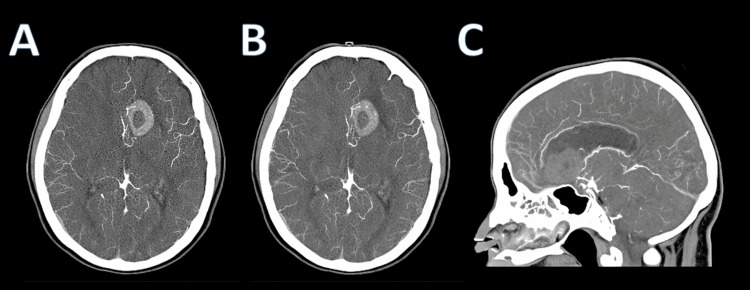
CTA of the head. (A, B) Axial CTA images showing the left frontal hemorrhagic lesion with adjacent vascular structures. (C) Sagittal CTA view demonstrating the vascular anatomy and absence of a focal malformation at the hemorrhage site. CTA, computed tomography angiography
